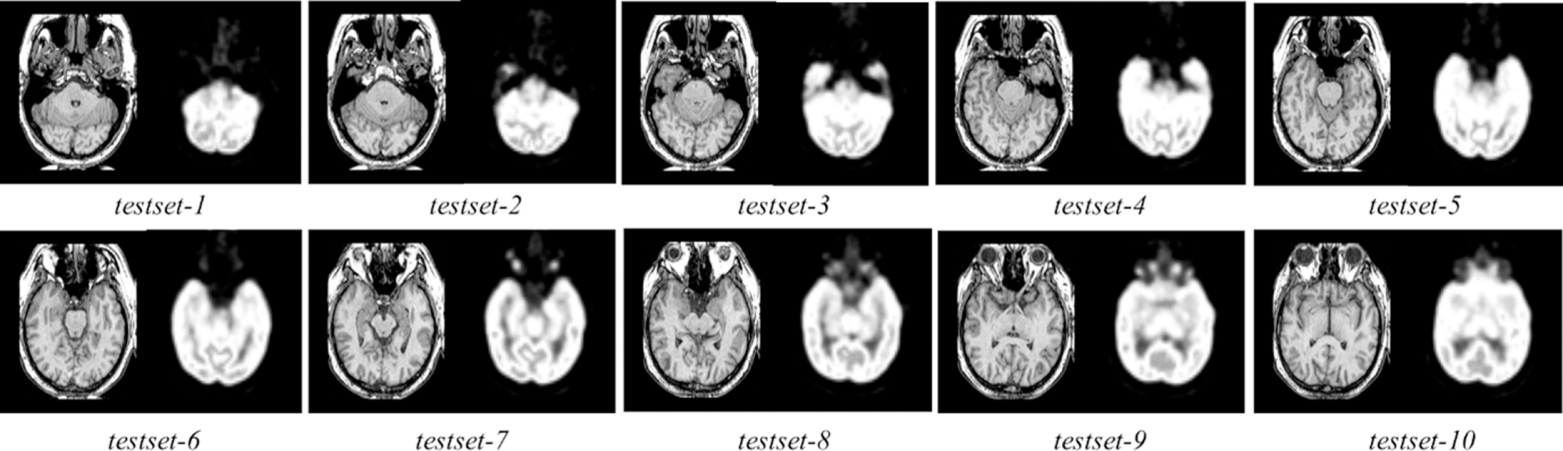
Evaluation dataset-1 for MRI-PET images.
